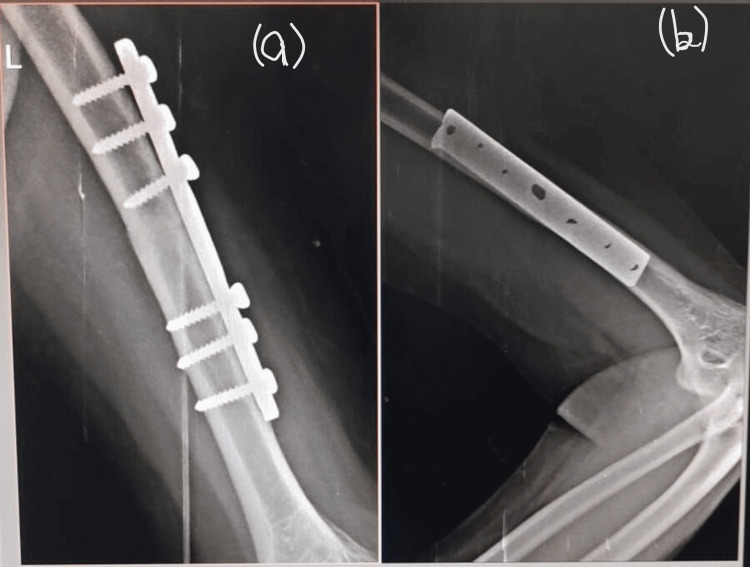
Radiograph showing fracture union, six months after the initiation of teriparatide (eight months from internal fixation) Radiograph of left humerus with plate in (a) anteroposterior view, (b) lateral view
